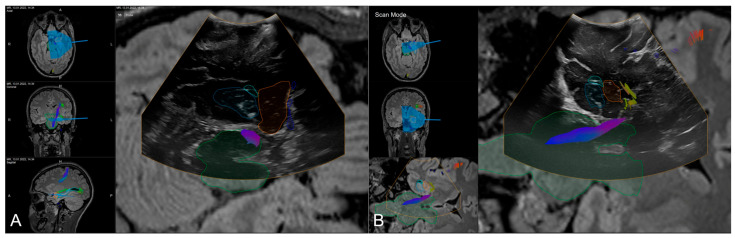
Navigated intraoperative ultrasound allowing for accuracy checks with outlined MRI-based objects (orange: amygdala, blue: hippocampus, green: brainstem, light blue: ventricle) and fiber tractography of the corticospinal tracts and optic radiation in axial (A) and coronar (B) view.
